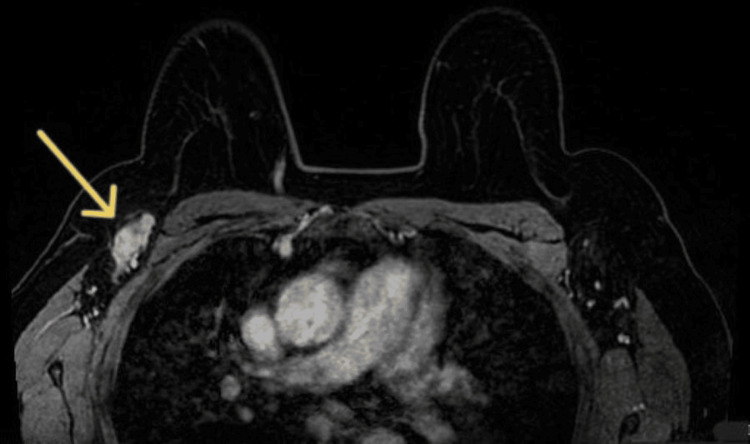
MRI showing suspicious 30 mm adenopathy (yellow arrow) in the right axilla.
